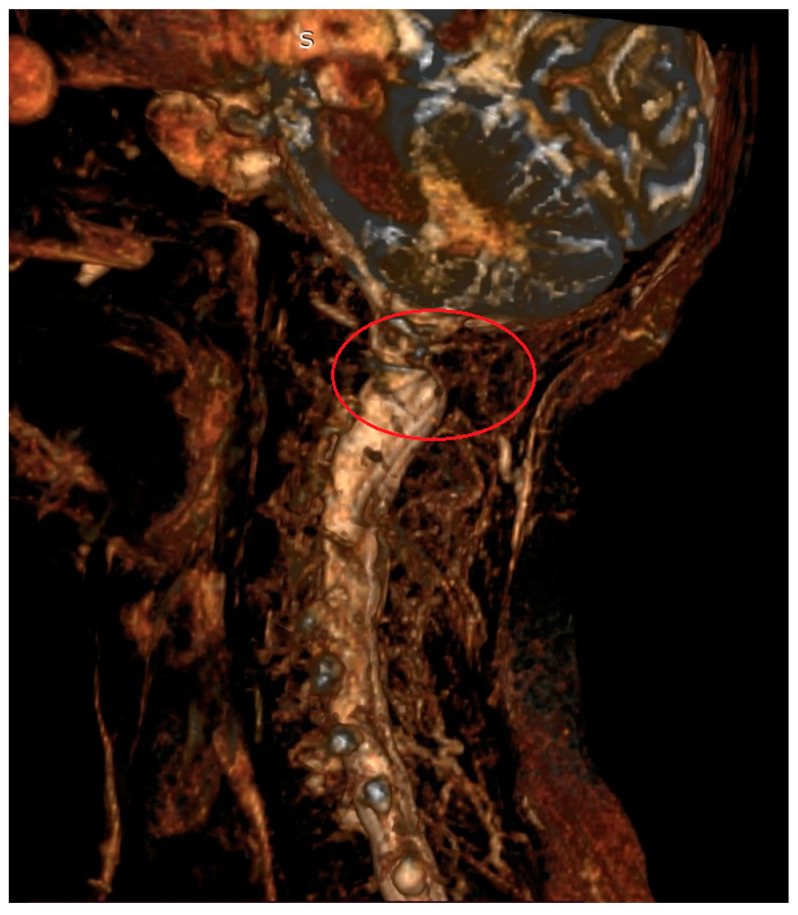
Magnetic resonance imaging (3D) of the cervical spine in a 67-year-old patient with AAS due to rheumatoid arthritis. Visible compression of neural structures in the area of the C1–C2 segment (red circle).
